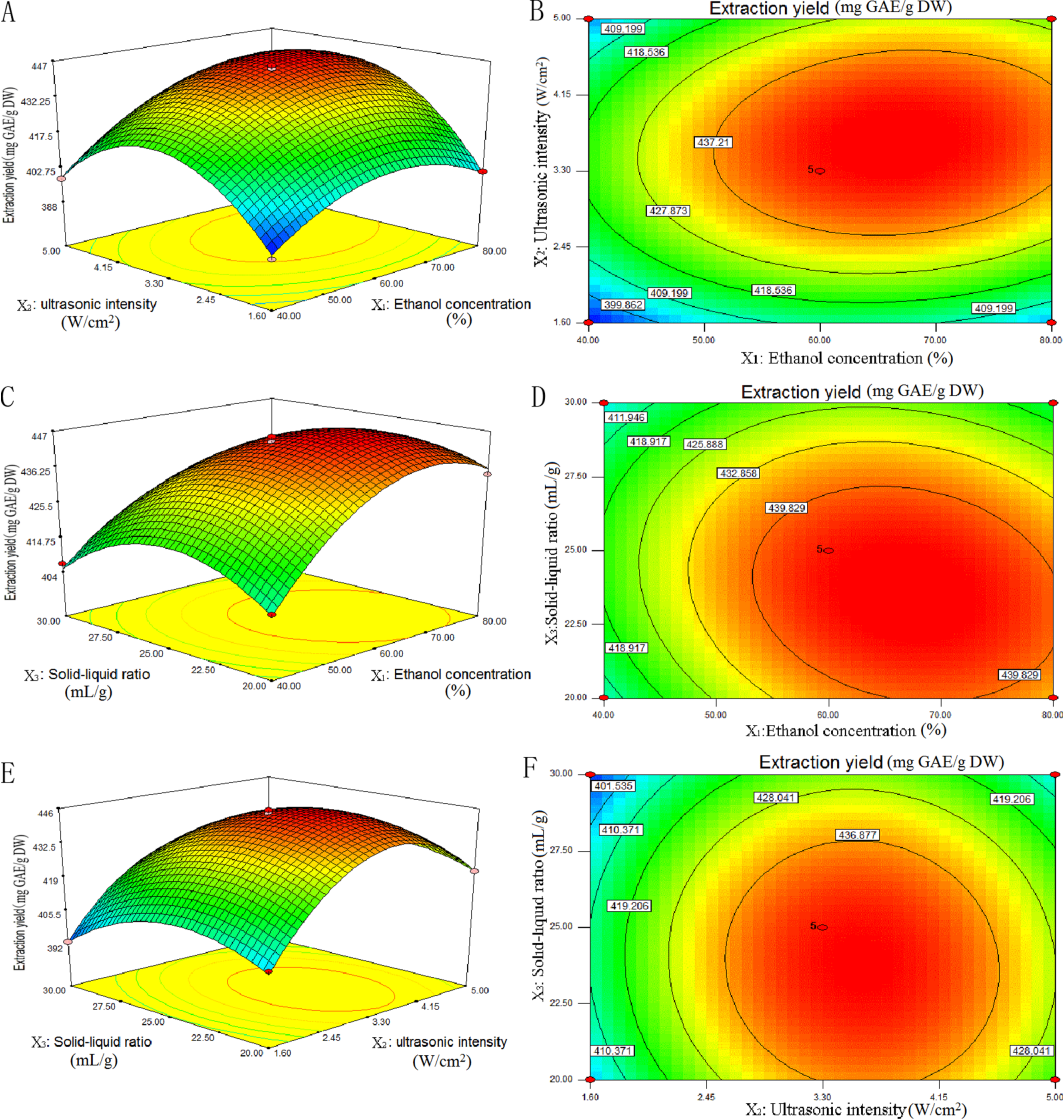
Response surface (3D) and contour plots (2D) showing the effects of different extraction parameters (X_1_: ethanol concentration, %; X_2_: ultrasonic intensity, W/cm^2^; X_3_: solid-liquid ratio, mg/mL) added on the response Y.
